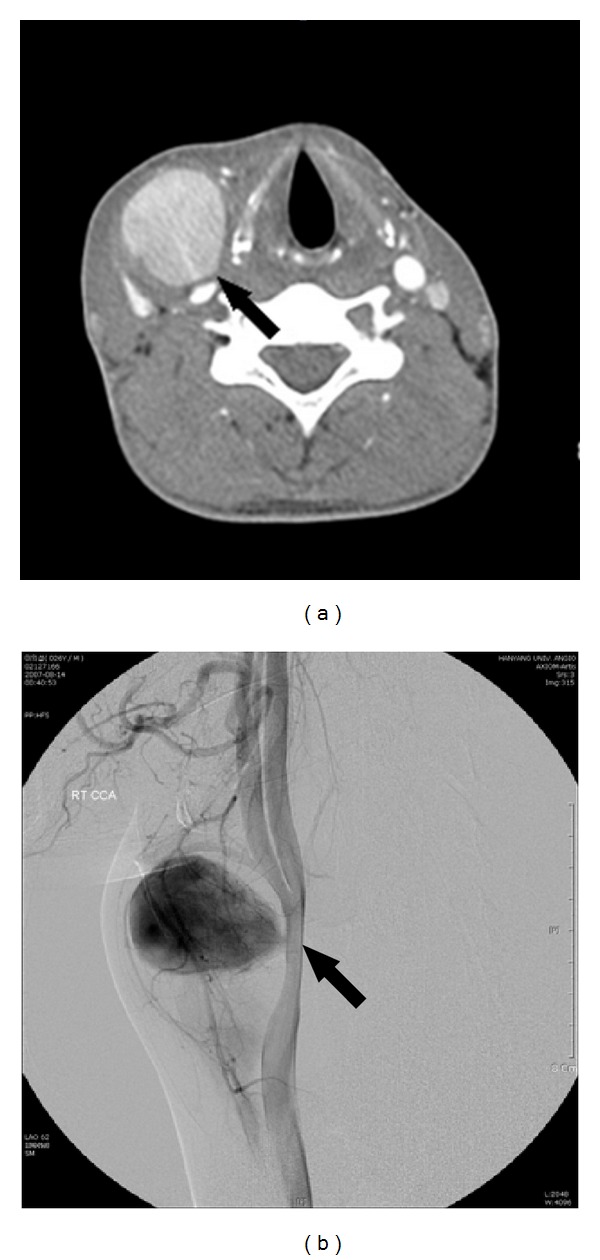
(a) Contrast-enhanced CT angiogram demonstrating a 3 × 3 cm sized pseudoaneurysm (arrow) at the right common carotid artery. (b) Right carotid angiogram demonstrating a pseudoaneurysm (arrow) arising from the right common carotid artery.
